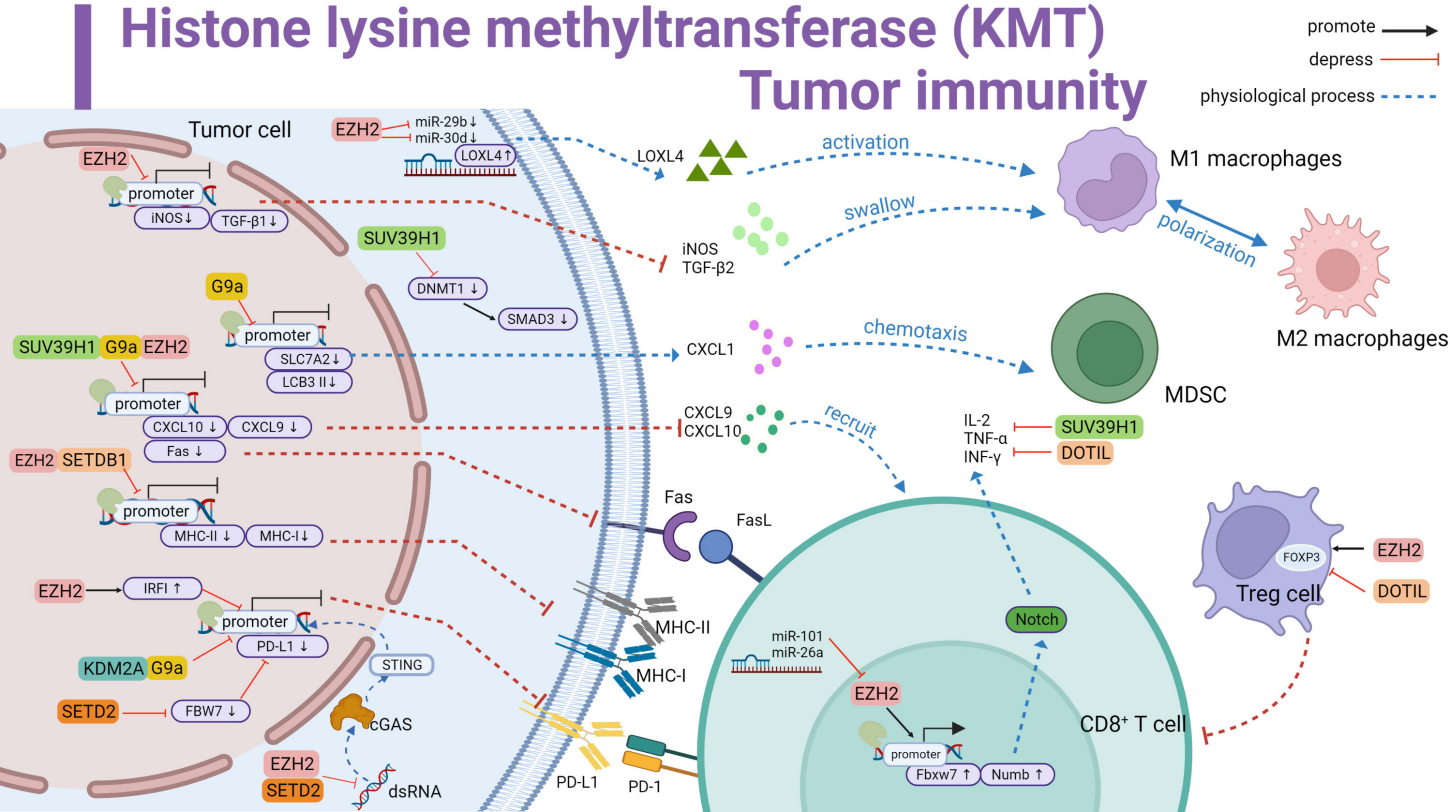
Histone lysine methyltransferase (KMT) involved in tumor immune summary. Promotes LOXL4 upregulation by antagonizing miR-29b and miR-30d to activate macrophage polarization; downregulates iNOS and TGF-β1 and inhibits macrophage phagocytosis. Downregulates iNOS and TGF-β1, inhibits macrophage phagocytosis. G9a inhibits SLC7A2, upregulates CXCL1 and thus recruits MDSC. G9a inhibits LCB 3II transcription and promotes immune escape. SUV39H1, G9a and EZH2 inhibit CXCL10 and CXCL9 transcription and reduce T cell recruitment. Meanwhile inhibit Fas transcription and curb Fas-FasL signaling pathway activation. EZH2 and SETDB1 repress MHC-II and MHC-I to affect antigen recognition. KDM2A and G9a directly repress the initiation of PD-L1 transcription. EZH2 and SETD2 inhibit the dsRNA-cGAS-STING pathway in the cytoplasm affecting PD-L1 transcription. In addition, EZH2 upregulates IRF1 to inhibit PD-L1 transcription. SETD2 downregulates FBW7 to inhibit PD-L1 expression. SUV39H1 inhibits SMAD3 in the cytoplasm and forms immunosuppression. EZH2 promotes FOXP3 transcription and Treg cell suppressor function. In contrast, DOTIL is the opposite. In T cells, EZH2 upregulates IL-2,TNF-αand INF-γ by promoting Fbxw7 and Numb activation of the Notch pathway, a process inhibited by miR-101 and miR-26a antagonism. SUV39H1 and DOTIL suppress the expression of immune factors. Black line represents promotion, red line represents inhibition, and dashed line represents physiological function.
